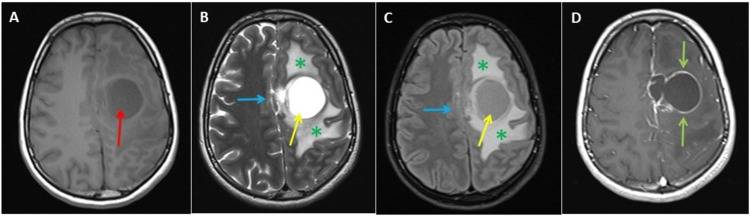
Magnetic resonance imaging (MRI) of the brain showing the cystic lesion and post-contrast images of the cystic lesion. MRI of the brain shows a large left frontal coalescent cystic lesion abutting the interhemispheric fissure. The cystic fluid was of mild T1 hyperintensity (panels A, red arrow), and transverse relaxation time/fluid-attenuated inversion recovery hyperintensity relative to the cerebrospinal fluid (panels B and C, yellow arrows). This lesion displays marked perilesional edema (panels B and C, green asterisks) and mass effect (panels B and C, blue arrows). Post-contrast T1 images display peripheral ring-like enhancement of the cyst wall (panel D, green arrows).
